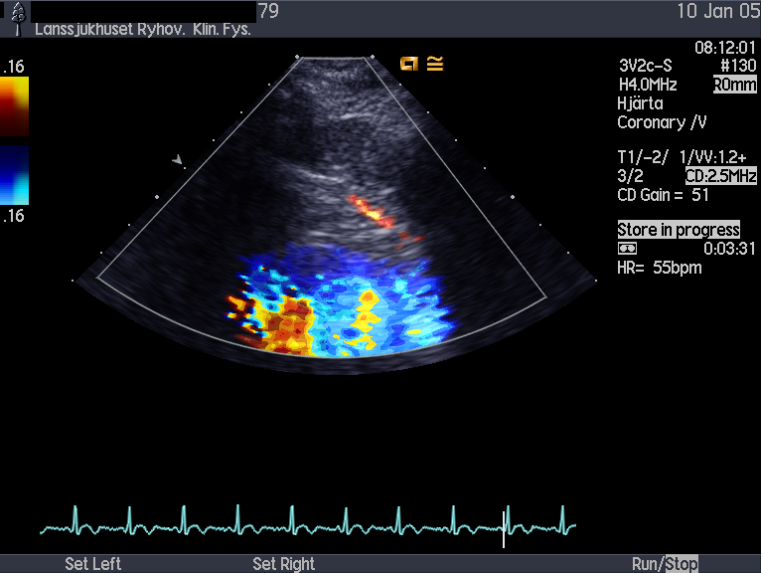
Colour Doppler recording of LAD flow at rest.
